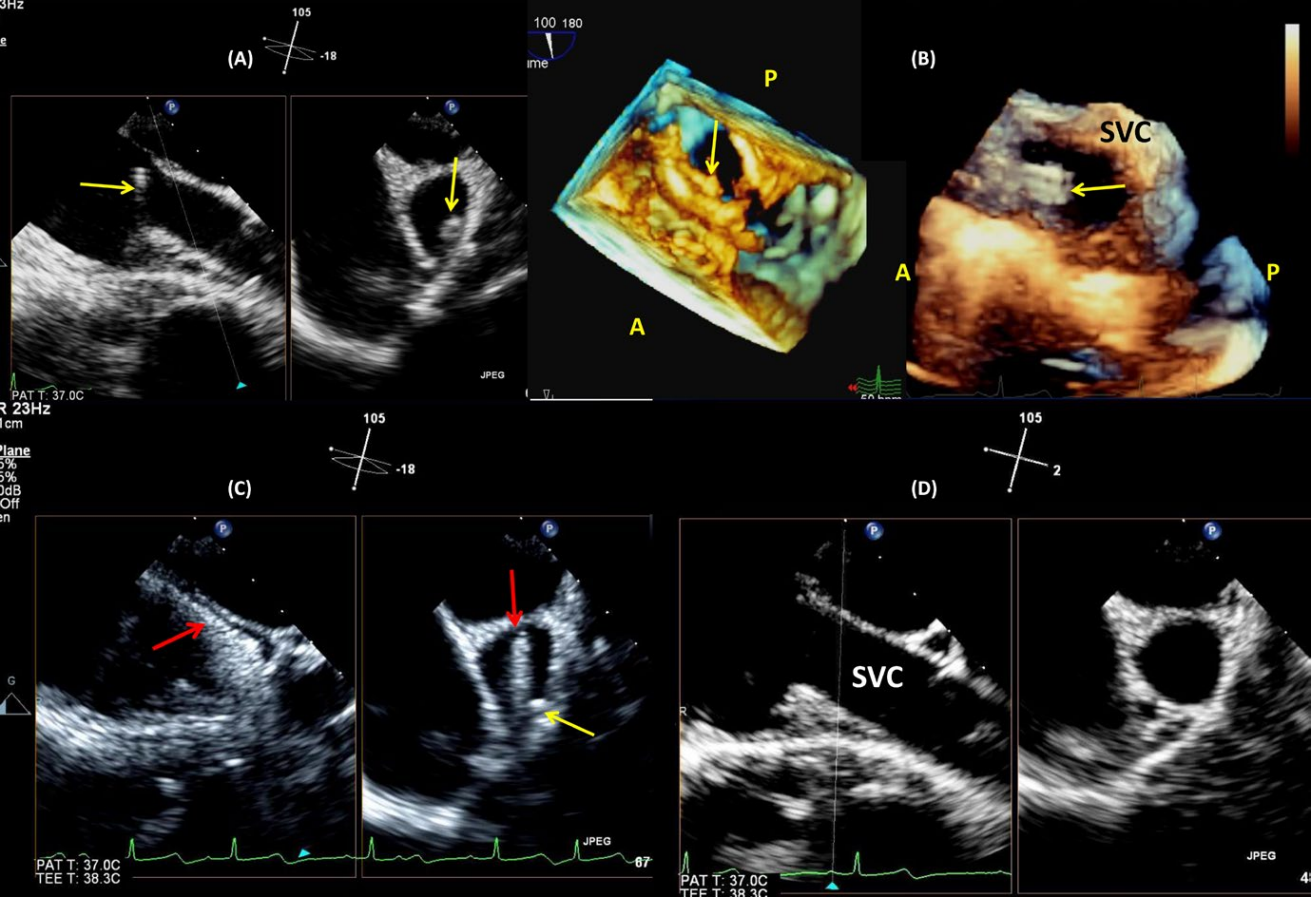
Multiplane/3D echocardiography guiding the extraction. A, biplane image of the superior vena cava (SVC), with the silicone sheath visible (arrow); B, 3D biplane images of the free upper end of the sheath in the last 4 cm of the superior vena cava; C, modified biplane bicaval view: the extraction tool (red arrow) and the free upper end of the sheath (yellow arrow) seen in the superior vena cava; D, following the procedure, the SVC is free of any echos
